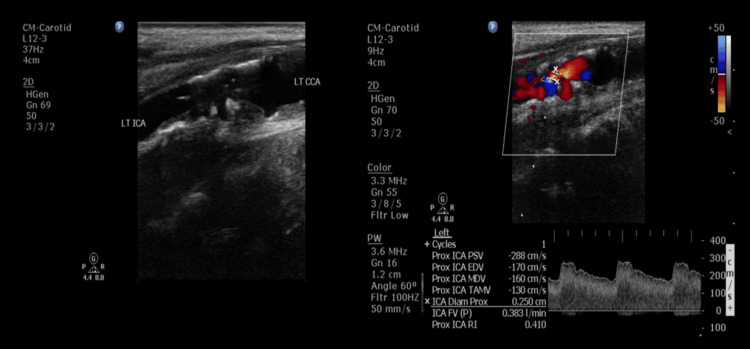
Carotid ultrasound May 2019 carotid sonography revealing atheromatous plaques with elevated mean flow velocities in the left proximal cervical ICA. ICA: internal carotid artery
